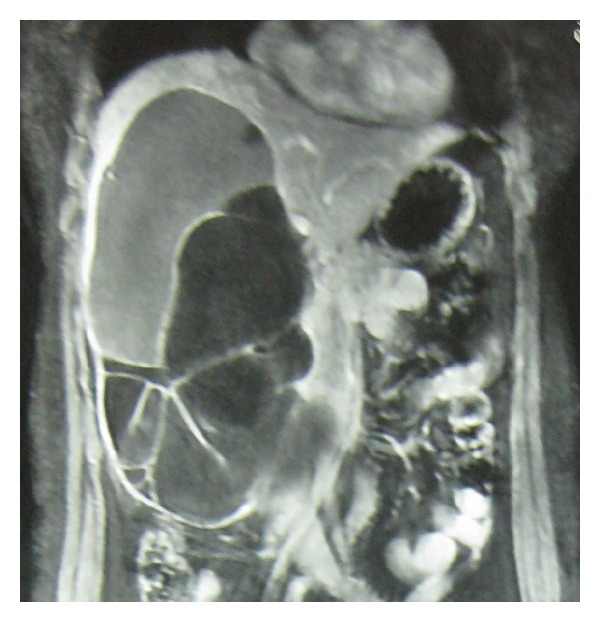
T1-weighted MRI image showing large multiseptated cystic lesion in right lobe of liver.
